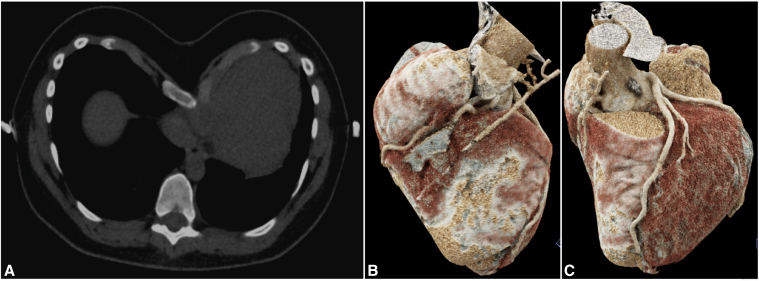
A, Preoperative axial computed tomography scan demonstrating marked cardiac displacement toward the left hemithorax due to severe pectus excavatum. B and C, Coronary computed tomography angiography showing normal coronary anatomy with no evidence of obstructive coronary artery disease or congenital anomalies.
